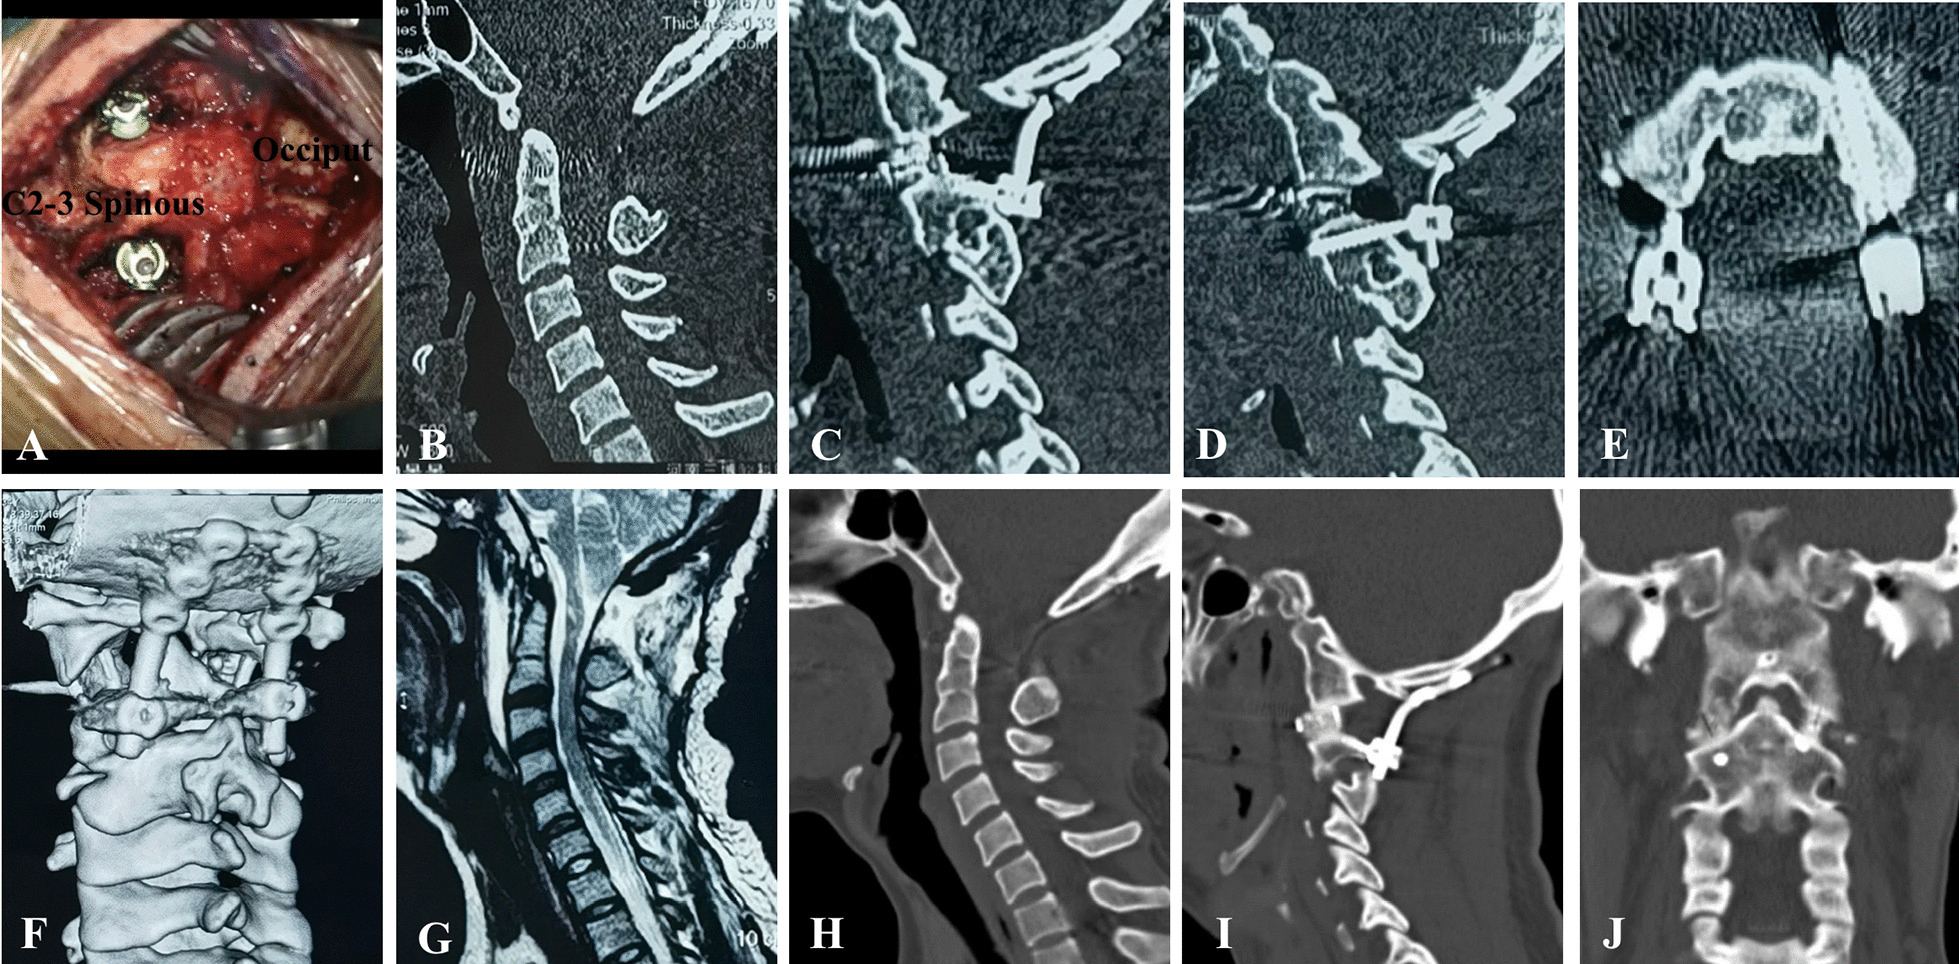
A After cage implantation, screws were inserted below the bilateral C2 articular surface. B Postoperative sagittal computed tomography (CT) showed that the odontoid process had been reduced and the cage was inserted anteriorly between the bilateral joints. C, D, E, F The insertion point of the C2 screw was located below the bilateral C2 articular surface and in a small area above the vertebral artery. The screw was inserted medially as far as possible. G Postoperative sagittal T2 magnetic resonance (MR) image showing that the ventral spinal cord compression was completely relieved, and the ventral spinal cerebrospinal fluid (CSF) signal was recovered. At 13 months after surgery, CT showed that the odontoid process remained in the reduced state (H) and the atlantoaxial interarticular bone bridge was formed (I, J)
